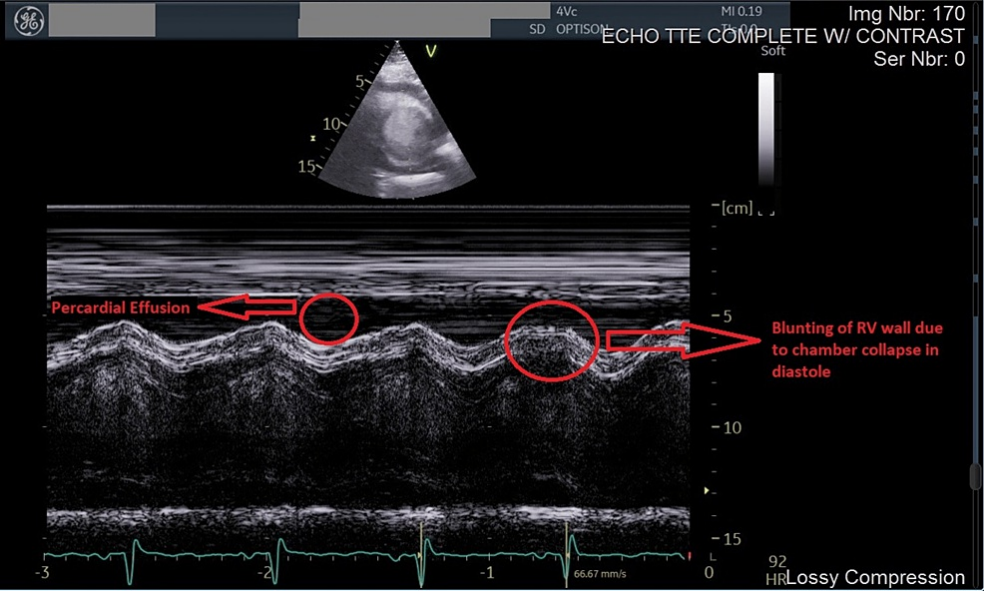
Right ventricular (RV) wall collapse during diastole on echocardiography
